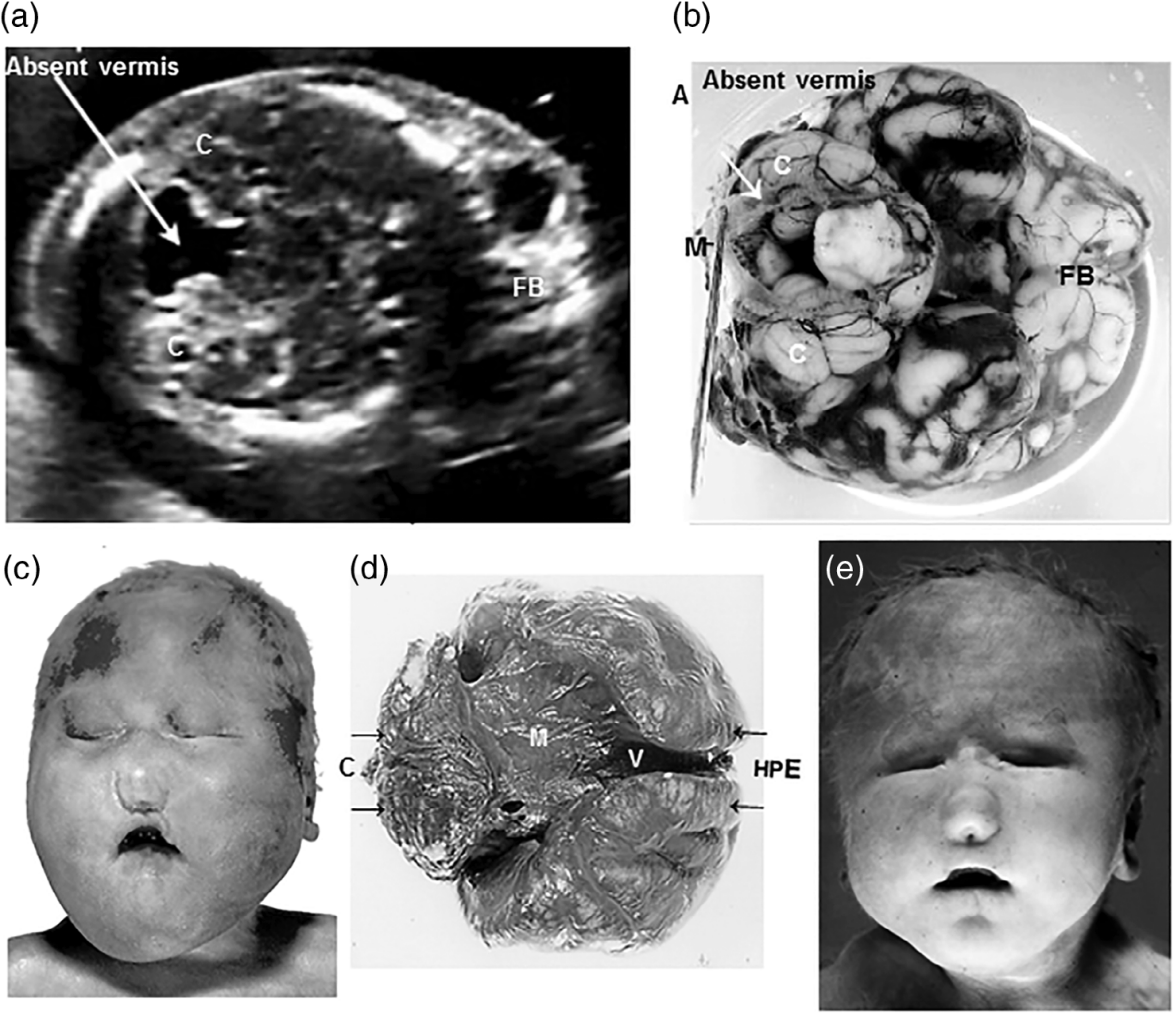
Agenesis of the cerebellar vermis with the remaining roofing membrane of the fourth ventricle distended to form a Dandy‐Walker cyst (DWC) in Case 7 as shown by prenatal ultrasound (a) and on a basal view of the brain at autopsy with the ruptured DWC on the left indicated by a lying probe (b). Facial aspects of the male siblings in Case 8 at 35 weeks (c) and Case 9 at 36 weeks (e) display cebocephaly with hypotelorism and a small nose with a single nostril. An occipital view of the brain of Case 8 shows lobar holoprosencephaly with a horseshoe‐shaped fused forebrain and dilatation of common cerebral ventricles, roofed by a collapsing membrane (d). FB, forebrain; HPE, holoprosencephalic forebrain; C, cerebellum; M, membrane, covering the DWC in Case 7 (b) and the common ventricular system in Case 8 (d)
